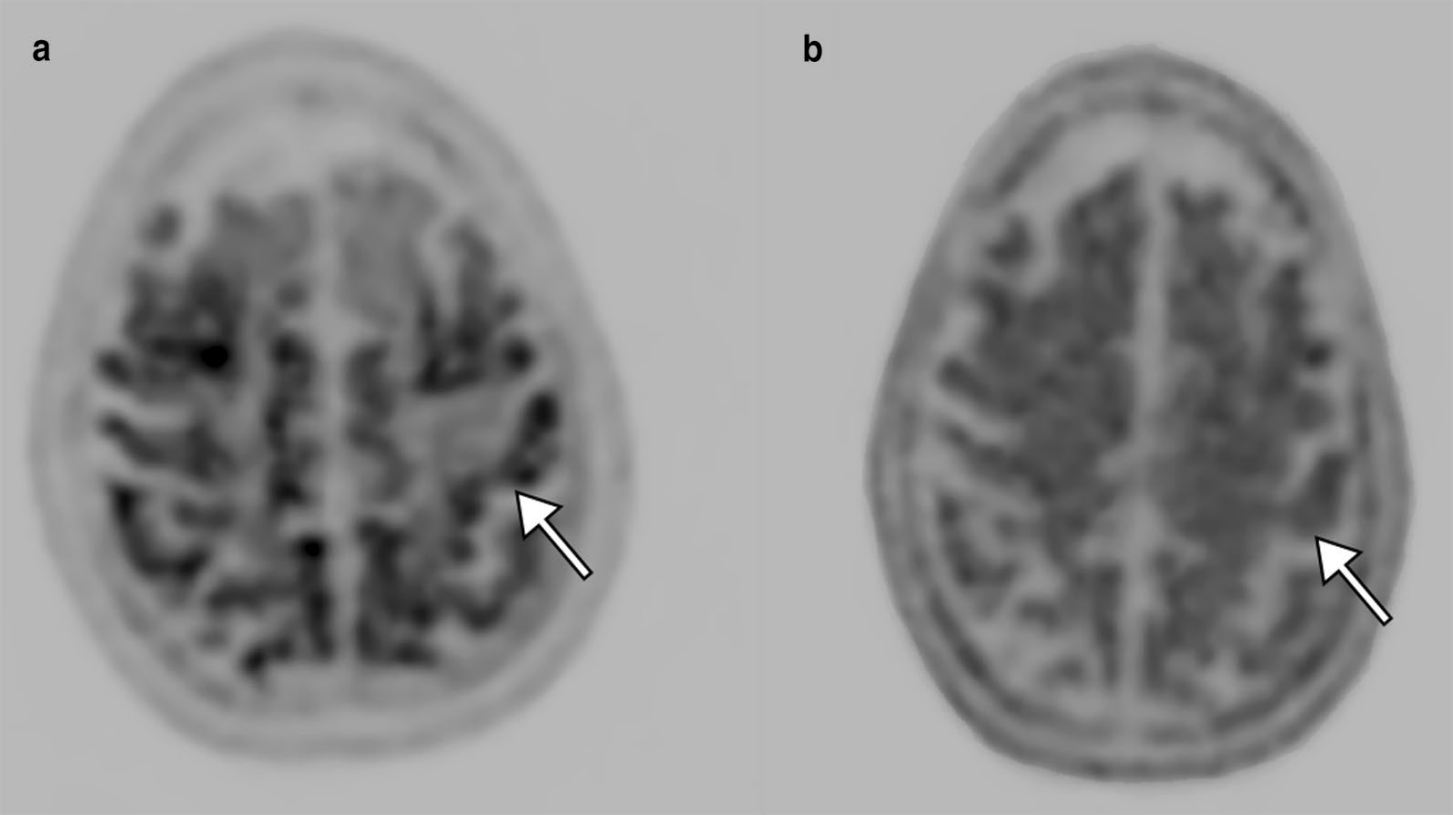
A 60-year-old female with cognitive decline (MoCA 19/30) and limb apraxia. Flortaucipir PET/CT (a) demonstrates tau retention in the bilateral perirolandic regions (arrow indicating left precentral gyrus). Florbetapir PET/CT (b) demonstrates radiotracer activity in the bilateral perirolandic regions (arrow indicating left precentral gyrus). Final diagnosis was CBS secondary to underlying AD, given the abnormal amyloid binding
